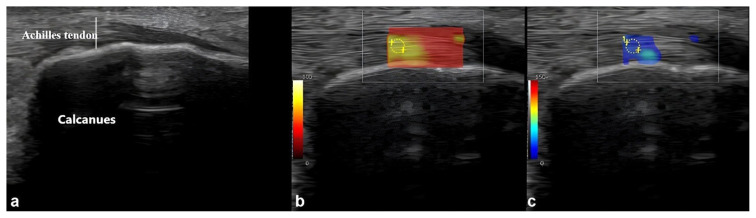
Ultrasonographic imaging sections showing measurements of the thickness (white line) (a), and stiffness (shear wave velocity) (b, c) of the Achilles tendon.
